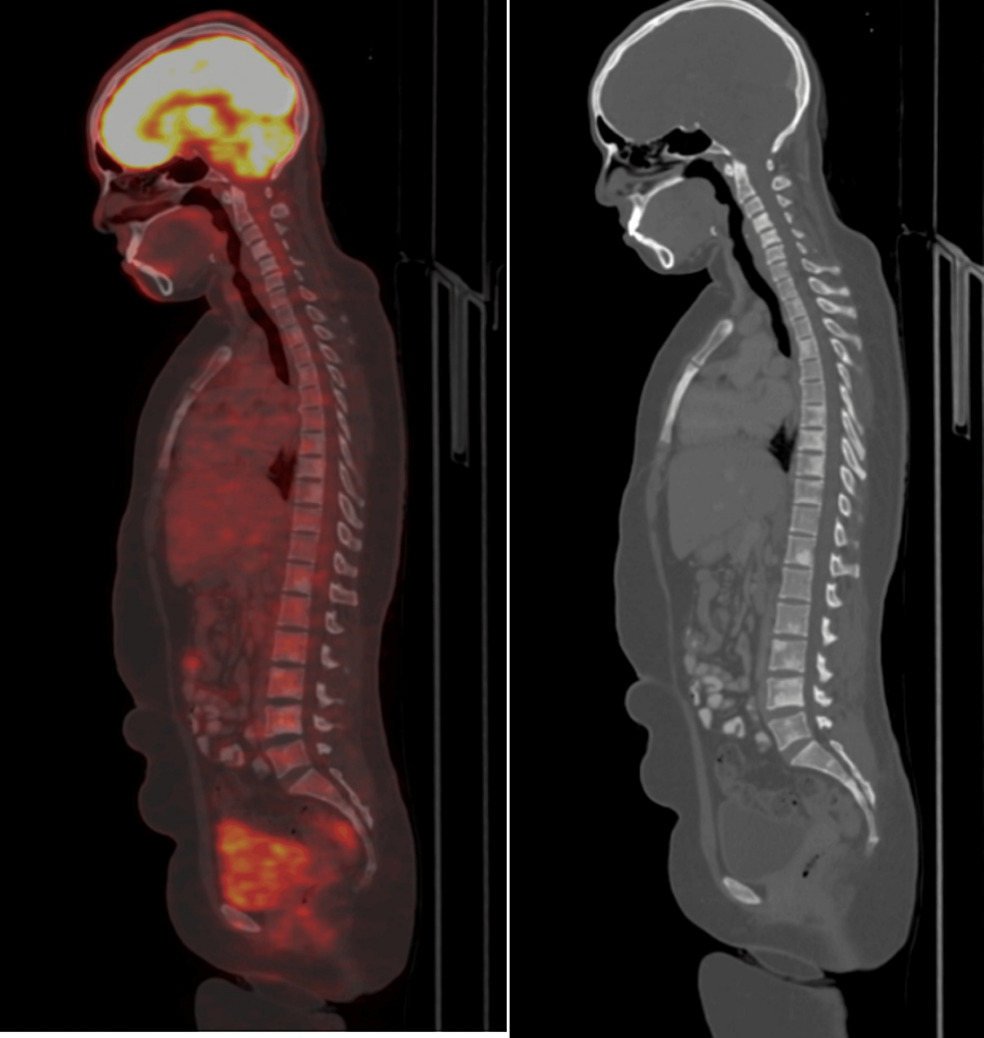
PET/CT images showing low-grade hypermetabolic uptake and lesions at multiple levels in vertebral bodies. PET: positron emission tomography
